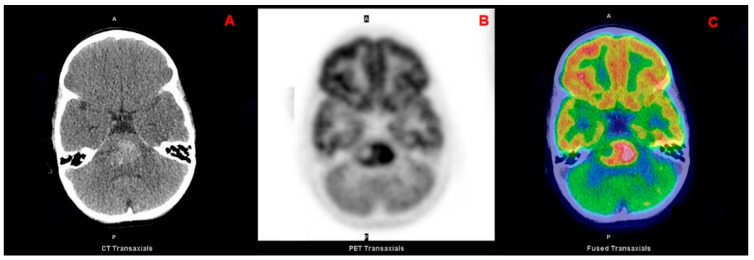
Transaxial CT (A), PET (B) and fused PET/CT (C) images of a pontine glioma.
